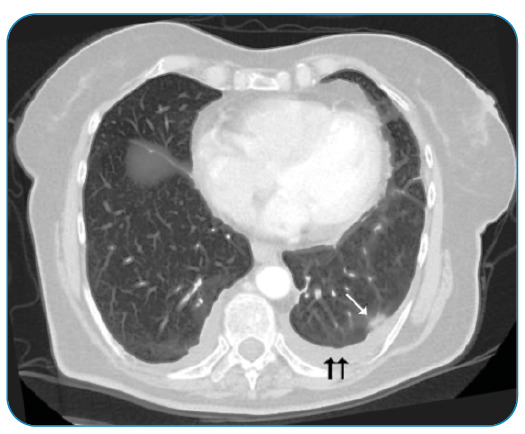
Thoracic computed tomography; Parenchymal nodules, ground-glass appearance (white arrow) and pleural effusion (black arrows) are seen in the lower lobe of the left lung.
